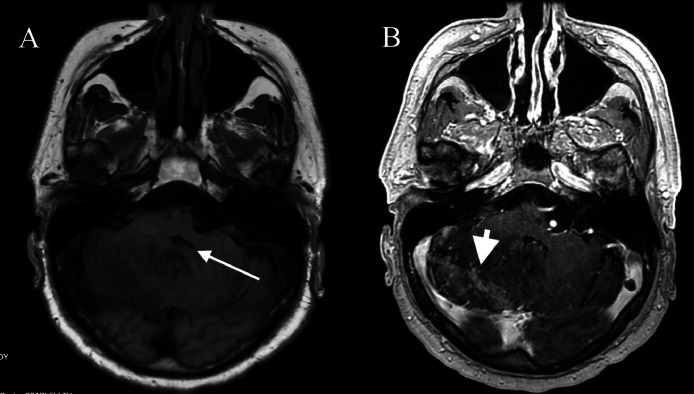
T1-weighted precontrast axial MRI brain (A) showed less marked hyperintensity in the right cerebellum, but worsening edema with almost complete effacement of the fourth ventricle (arrow). T1-weight postcontrast axial MRI brain (B) showed similar patchy enhancement within the area of edema (arrowhead), without obvious mass lesion.
